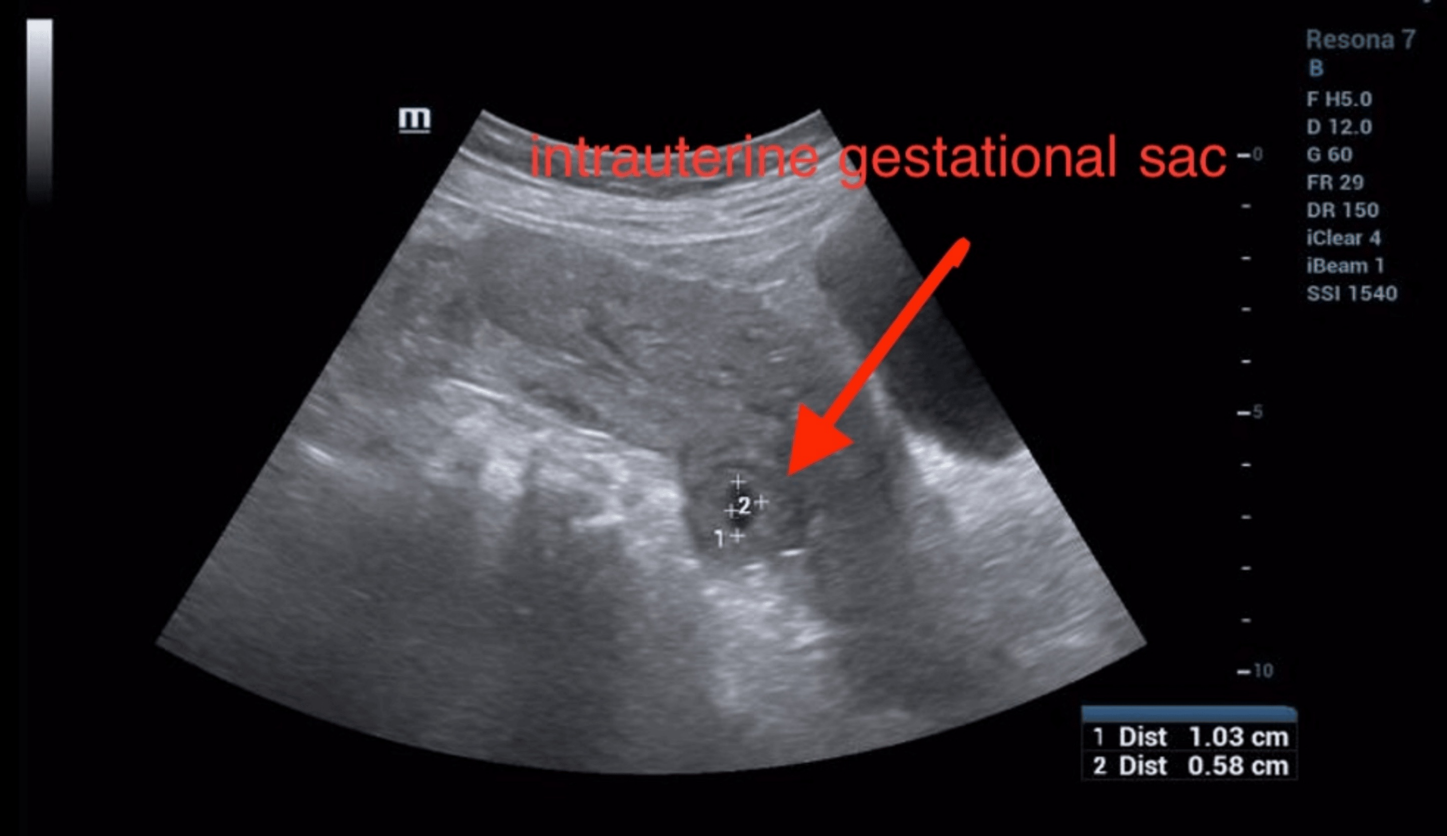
Transabdominal ultrasonography image of uterus shows a single empty gestational sac seen in the uterine cavity.
